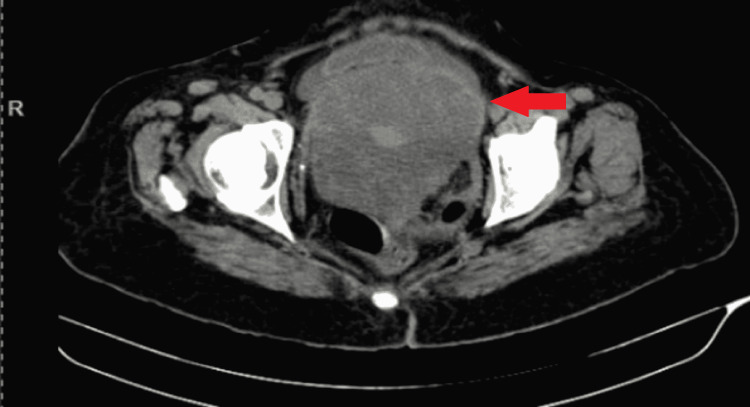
A contrast-enhanced CT scan revealed a large heterogeneously enhancing mass lesion in abdominopelvic region CT: Computed tomography
